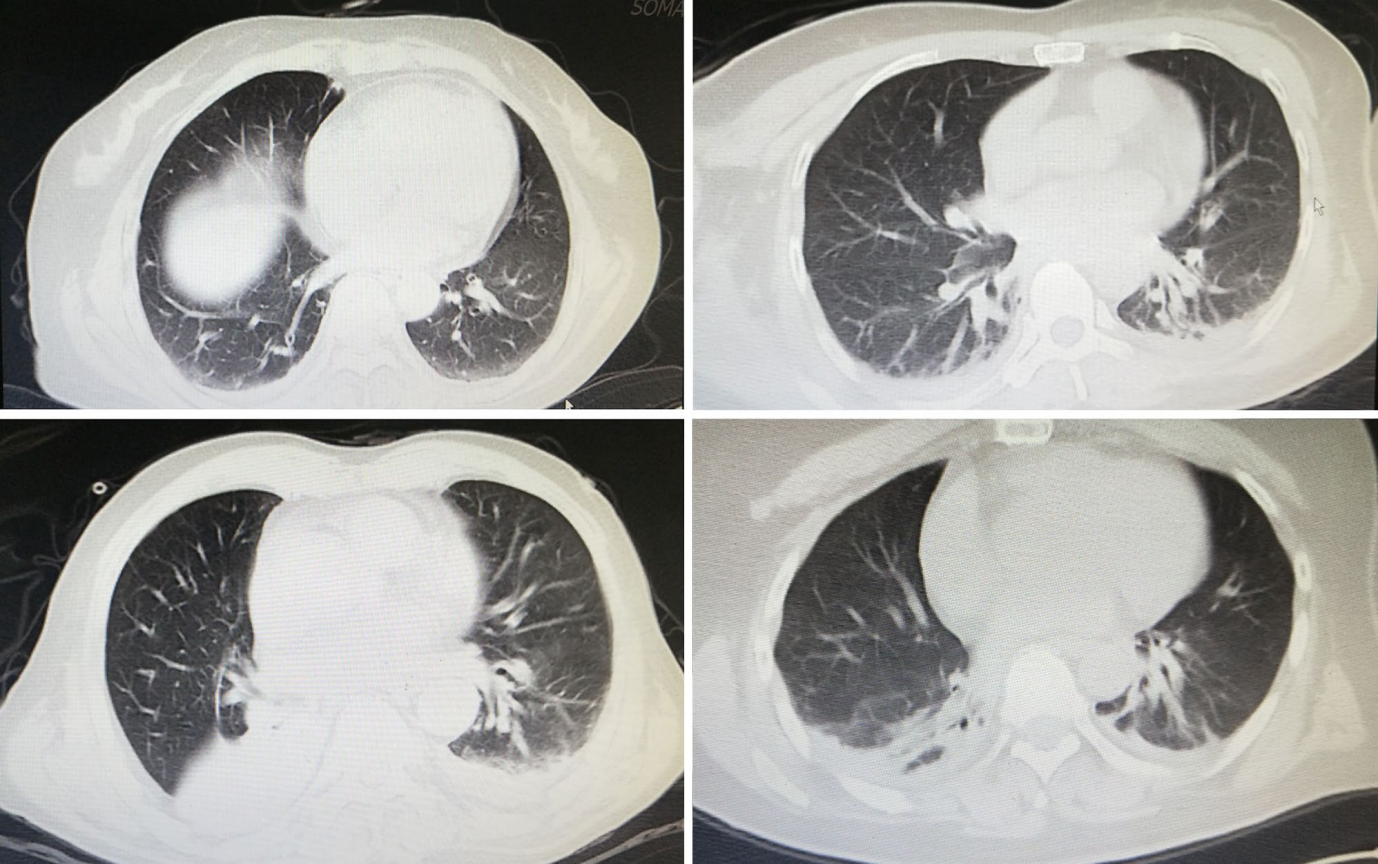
The first postoperative chest CT image showed bilateral pulmonary imaging abnormalities after craniotomy of brain tumor.
